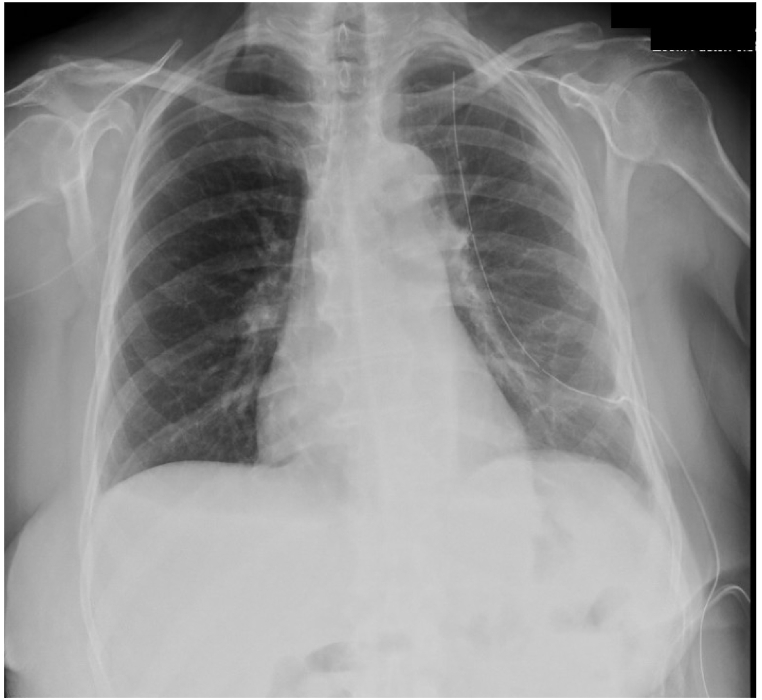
Chest radiograph just prior to TPC removal, five-months after initial placement, demonstrating small residual effusion.
